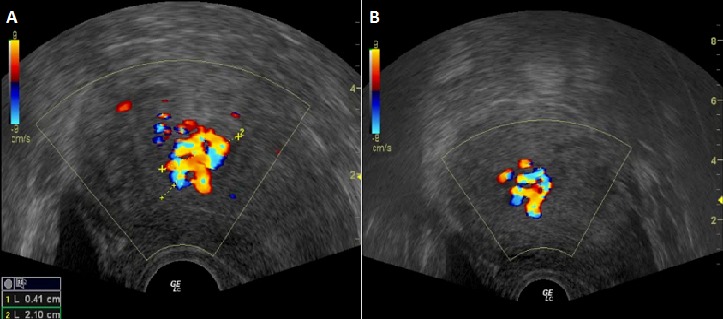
Échographie pelvienne avec doppler couleur objectivant une invasion du myométre sur le mur antérieur de l'utérus
